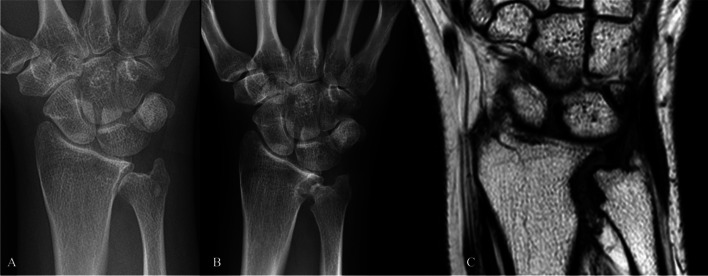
PA radiographs in case 2 showing a reduced joint space in the DRUJ preoperatively (A). At 1 year follow-up, some of the subchondral bone appears uneven but the distance between radius and ulna is wide (B). MRI 2 years postoperatively revealed similar findings (C)
